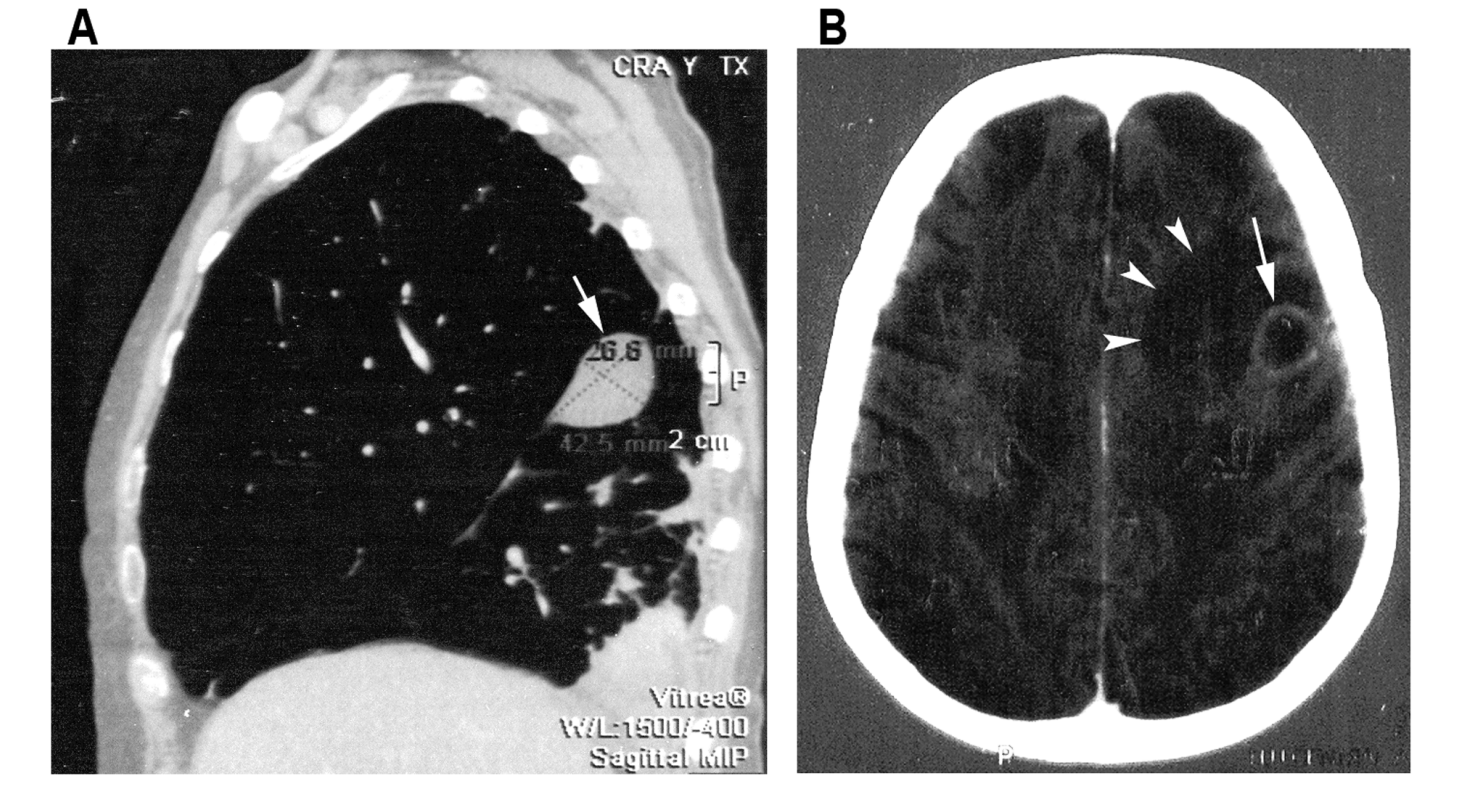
(A) Computed tomography of the chest showing multiple pulmonary nodules, a small collection of fluid in the major fissure (arrow) and a small pleural effusion on the right. (B) Computed tomography of the brain showing a nodular lesion in the left frontoparietal lobe (arrow) with perilesional vasogenic edema (arrowheads).
